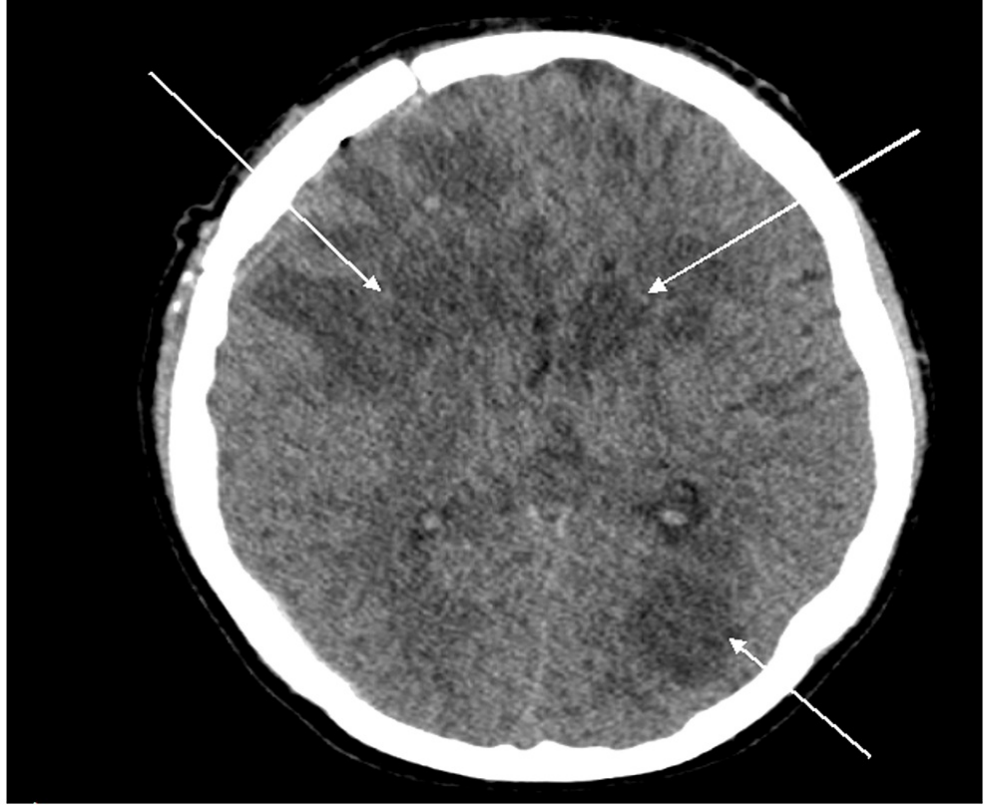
Post-craniotomy computed tomography scan of the brain showing partial evacuation of the right frontal lobe lesion and multifocal hypodense lesions as shown by arrows in both cerebral hemispheres
